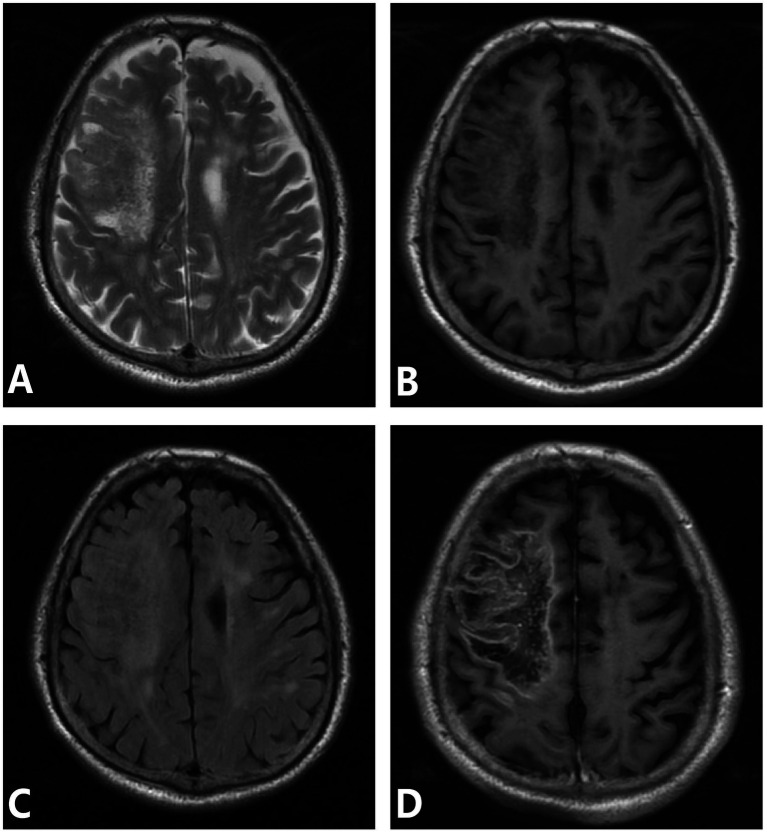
(A–D) Meningovascular type neurosyphilis, a 65-year-old male presented with episodic limb twitching for 1 month and recurrence with left limb weakness for more than half a month. MRI showed abnormal lesions in the right frontoparietal lobe, which showed low signal intensity on T1WI, heterogeneous high signal intensity on T2WI and FLAIR, and gyral and geographic enhancement on enhancement. Panel (A) (T2WI): shows heterogeneous high signal intensity; panel (B) (T1WI): shows low signal intensity in the right frontoparietal lobe; panel (C) (FLAIR): depicts hyperintense lesions with edema; panel (D) (T1WI-CE): demonstrates gyral and geographic enhancement.
